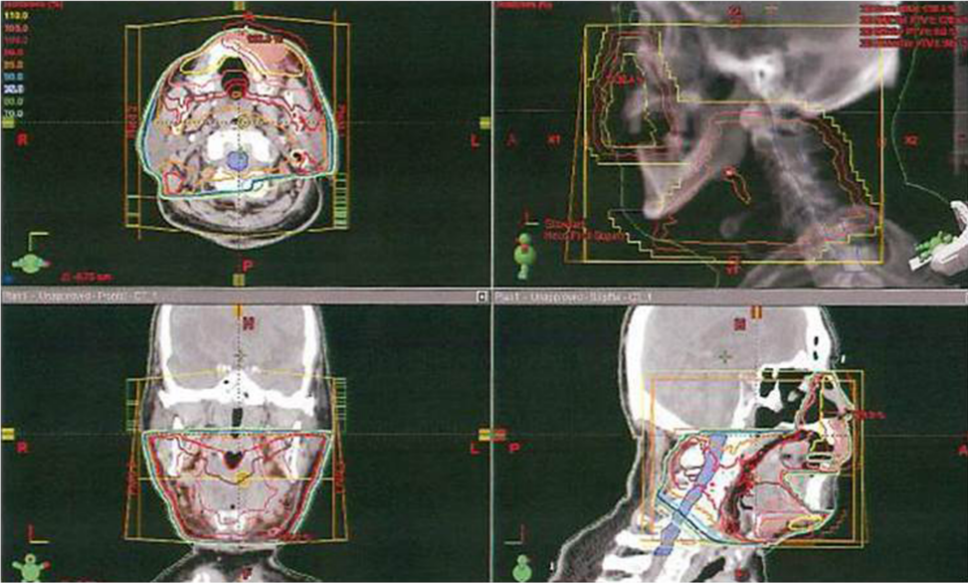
Field settings for radiotherapy. The patient received 4-MV linac X-ray, lateral parallel opposed paired ports for primary site and bilateral levels I and II (45 Gy/25 fr) plus electrons for the primary site alone (20 Gy/10 fr). Field margins were ≥2 cm around the lesion.
